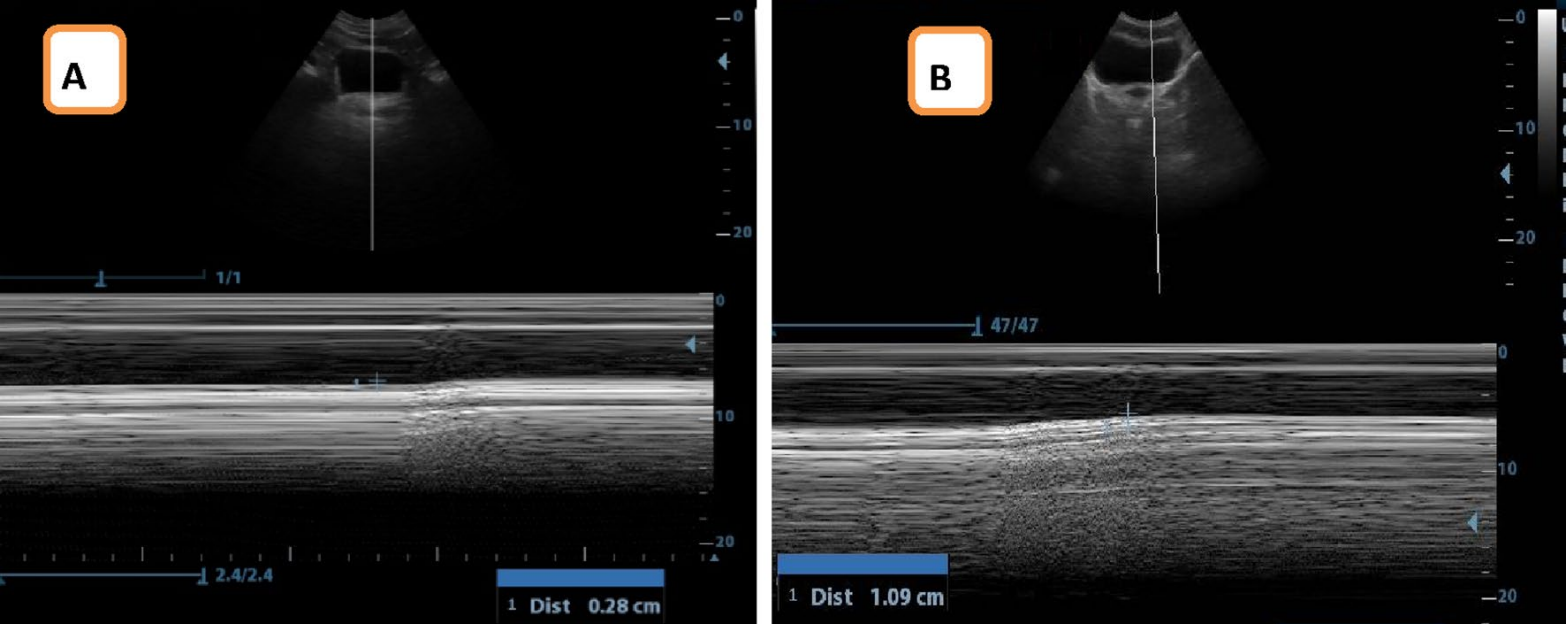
Ultrasound measurement of PFM displacement (A) VL group, (B) control group.
